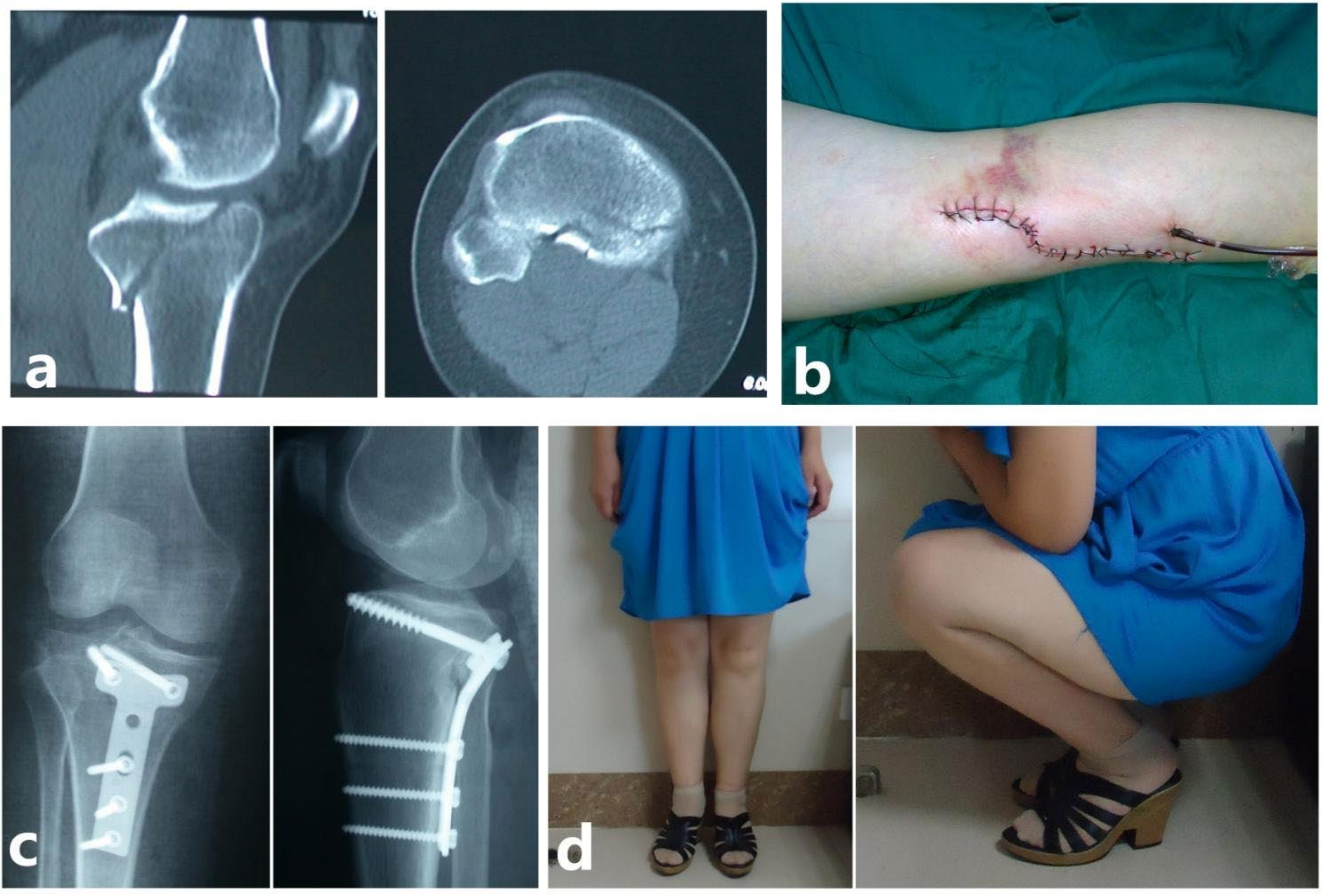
A typical case of a 44-year-old female with Schatzker IV tibial plateau fractures (flexion-varus-external rotation type). (a) The features of this type were shown in CT images. (b) Carlson Approach was needed for the reduction of MFA. (c) After the reduction of MFA, a plate should be placed upon MFA to fix the posteromedial fragment. (d) 1 year postoperatively, the function of the knee was excellent
